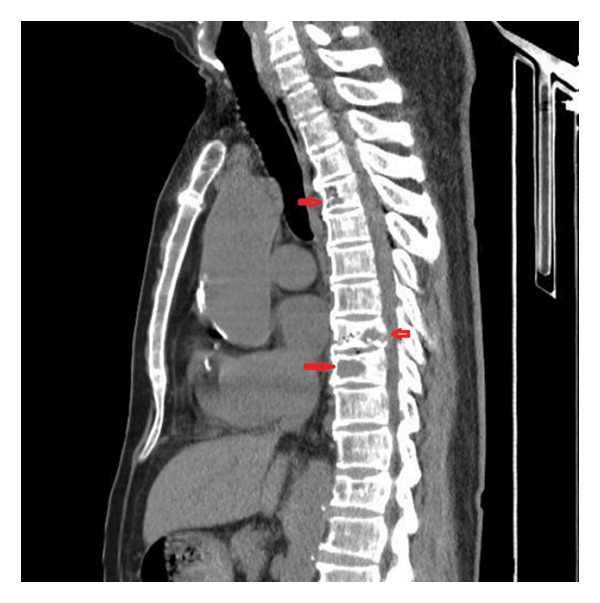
Computed tomography showing multiple osteolytic lesions.
